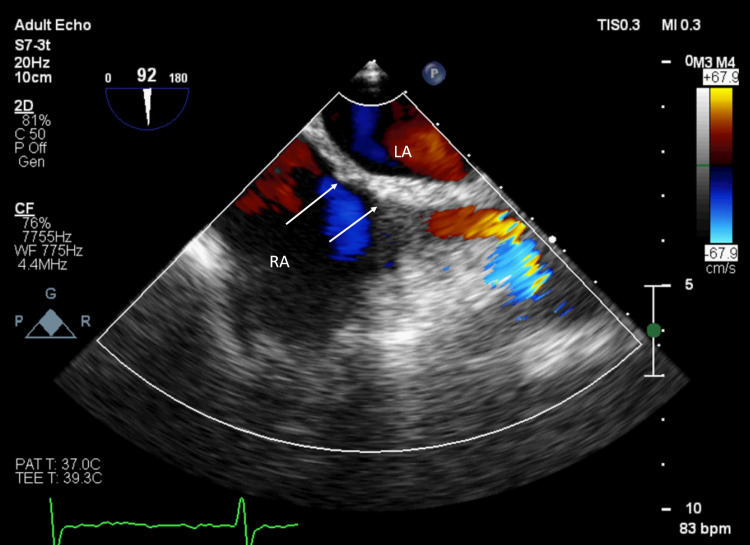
Transesophageal bicaval view. White arrows show an intact patch across the secundum atrial septal defect. LA: left atrium, RA: right atrium.
